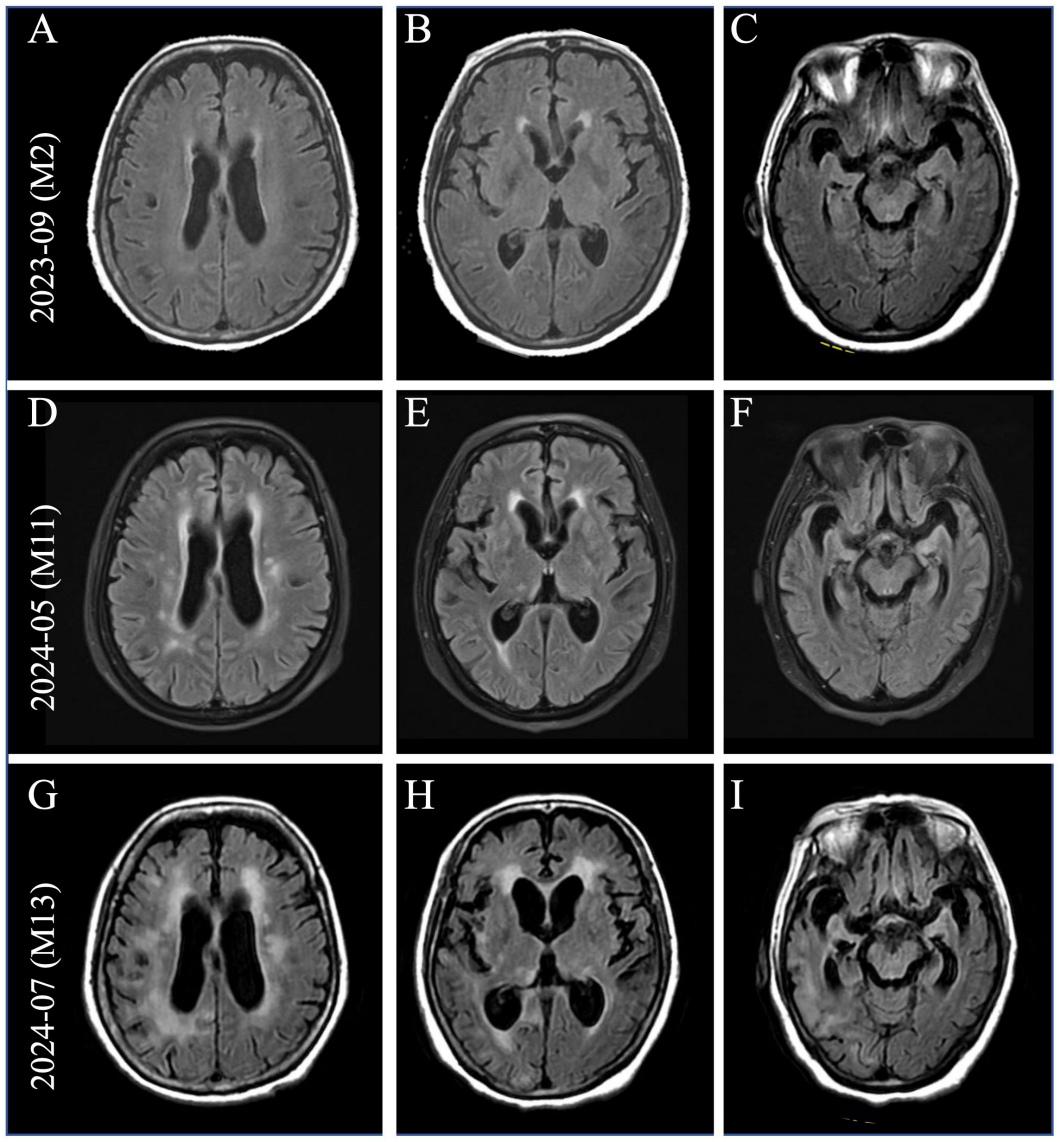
Brain imaging at the time of clinical progression. (A–C) Brain MRI (pictured: axial T2 fluid-attenuated inversion recovery) showed non-specific white matter changes two months after onset. With the development of severe neurological symptoms, brain MRI showed gradually increasing white matter hyperintensities (WMHs) in the periventricular regions (D, G), bilateral basal ganglia (E, H), and progressive brain atrophy (F, I).
